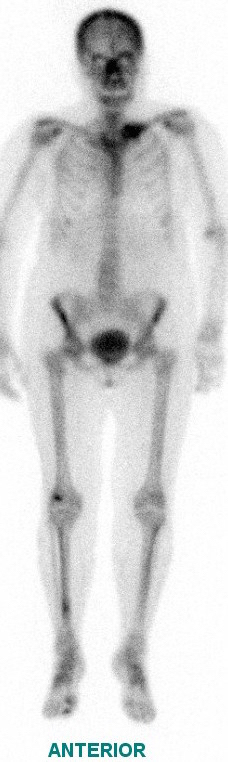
Bone scintigraphy showing an area of increased activity in the left clavicle correlating with the CT findings. An area of increased activity was also noted in the distal shaft of the right tibia and was suspicious of metastatic disease. This area, however, was later proven to be a false positive.
